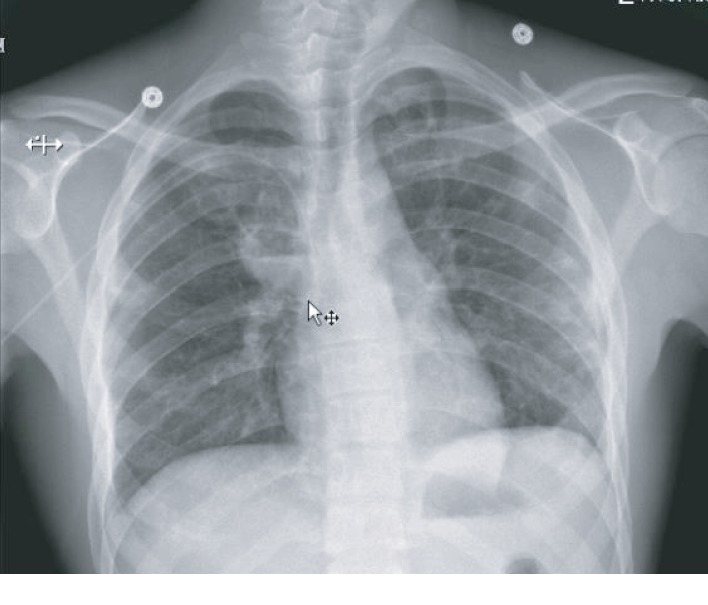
Chest X-ray showing interval improvement of left chylothorax 10 days after intrapleural fibrinolytics and diet modification.
